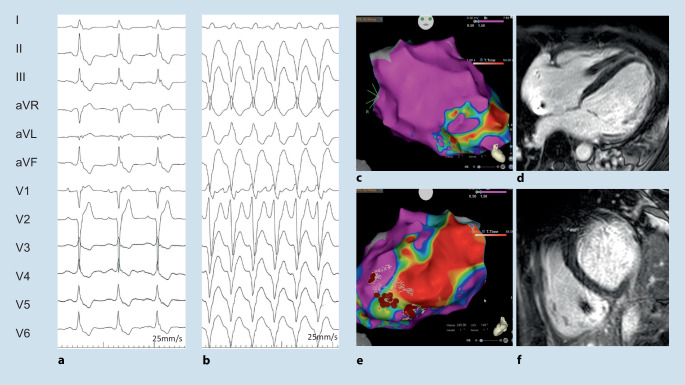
A 51-year-old male with genetically proven Anderson–Fabry disease and a history of aborted sudden cardiac death 4 years before presentation. After initial diagnosis of an assumed viral myocarditis, the patient presented 4 years later to the authors’ department with recurrent ventricular tachycardia (VT) (b). Late-stage Fabry disease with cardiac, neurological, and renal impairment was diagnosed. Cardiac magnetic resonance imaging (d,f) demonstrated apical and extensive posterolateral scarring that correlated to electroanatomical bipolar CARTO maps (c,e). The patient underwent endo- and epicardial VT ablation
